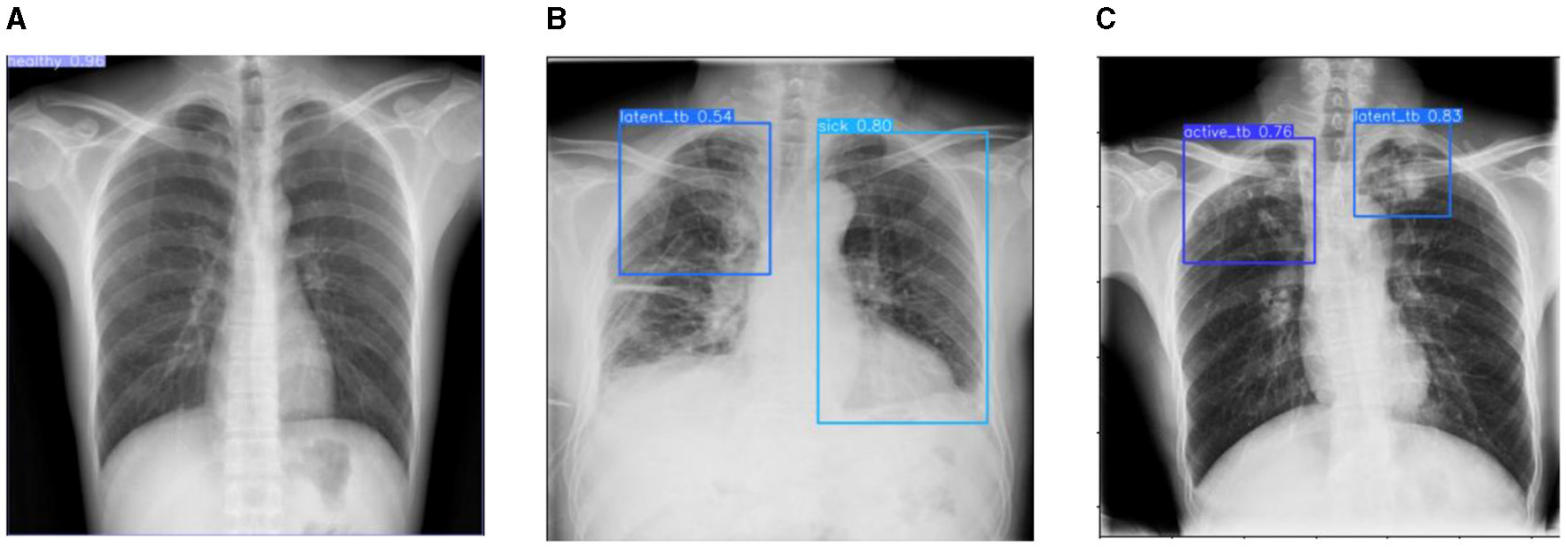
Prediction results of the proposed YOLOv8 model. (A) X-ray with healthy lung. (B) X-ray with sick region. (C) X-ray with latent TB.
